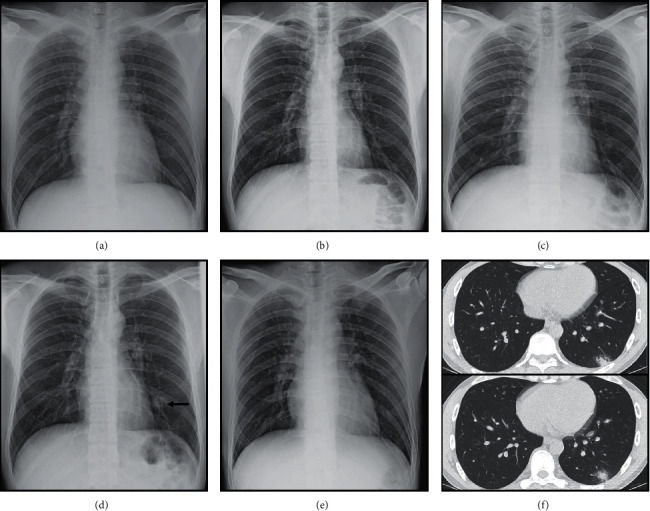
Images in a 37-year-old male patient with acute myeloid leukemia whose serum galactomannan test was positive. (a) Baseline chest film shows no opacity. (b) Chest film on first day of febrile neutropenia (ANC = 11 *μ*L) shows no opacity. (c) Chest film on third day of febrile neutropenia (ANC = 17 *μ*L) still shows no opacity. (d) Chest film on fourth day of febrile neutropenia (ANC = 0 *μ*L) shows new opacity at left lower lung (arrow). (e) Chest film on seventh day of febrile neutropenia (ANC = 2511 *μ*L) shows improvement of lower lung opacity. (f) Axial CT chest on the fourth day of febrile neutropenia reveals a nodular-like consolidation with surrounding ground-glass attenuation, representing a CT halo sign. This case was evaluated as true-positive chest radiograph and CT chest.
